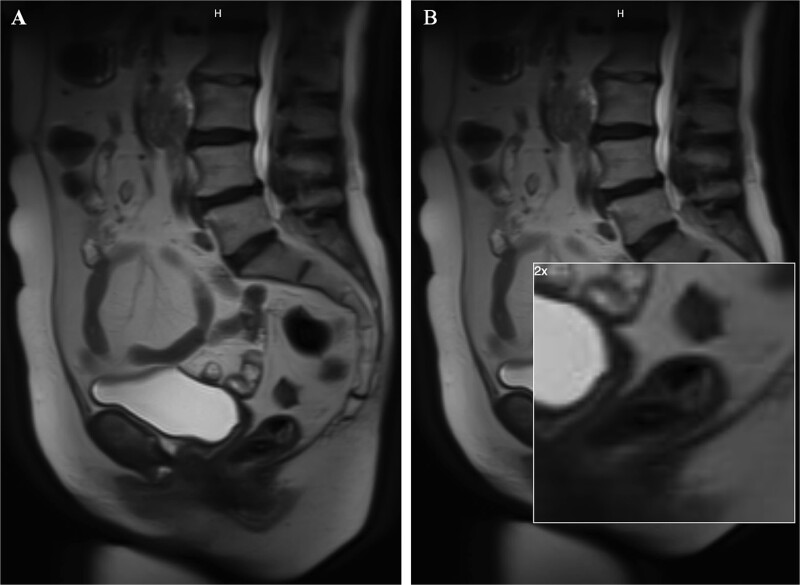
Images from the MRI abdomen pelvis with contrast. This depicts a small polypoid enhancing 2.2 cm mass within the posterior wall of the left mid rectum without invasion of the muscularis propria. The image on the right is a zoomed in image of the remaining polyp.
